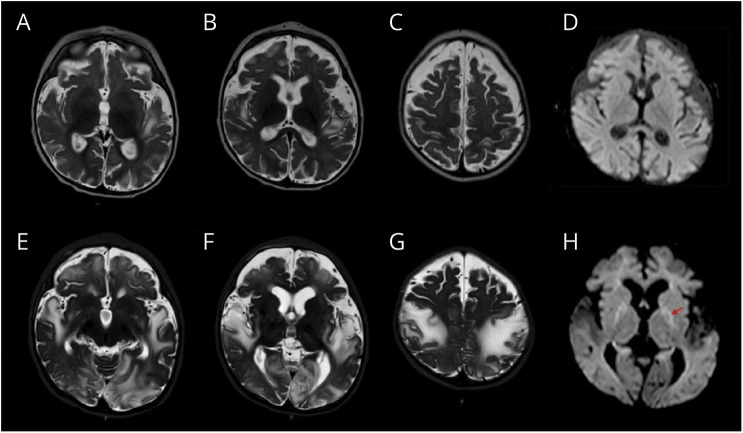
Brain MRI Study (A–D) T2-weighted images showing high-intensity signal in the insular and postcentral gyrus areas and sub/cortical atrophy at 8 months, (E–H) follow-up study at 10 months showing severe worsening of signal alterations over time, with spread to the basal ganglia, temporo-occipital and frontal regions and progression of the sub/cortical atrophy over time.
